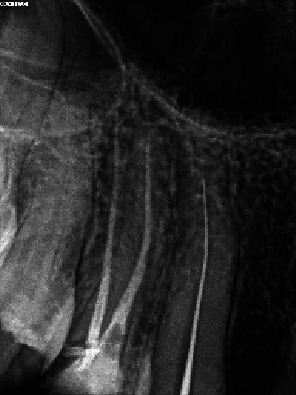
Periapical radiograph indicating a working length of 40 mm and the need for an additional 9 mm to achieve full working length.
